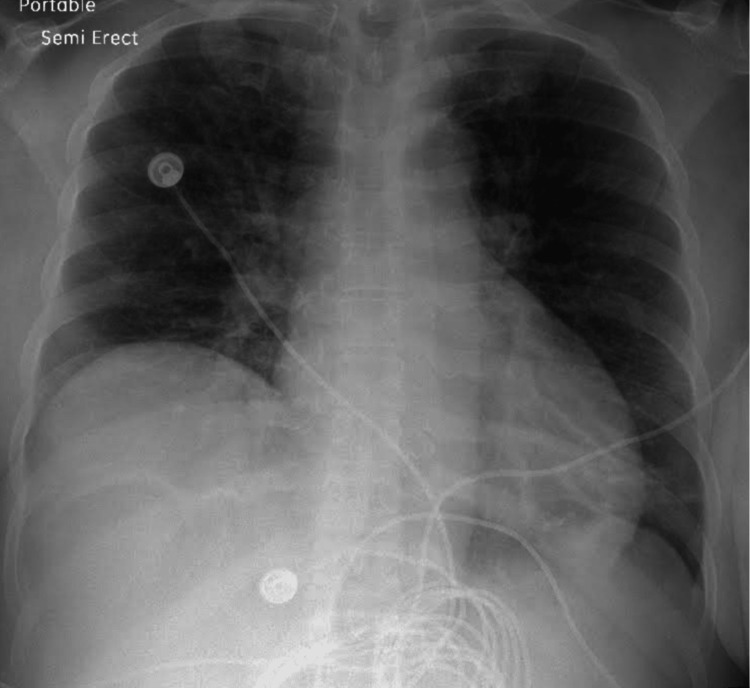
The initial chest x-ray depicting right upper lobar and diffuse left lung airspace opacities, possibly suggesting atypical pneumonia.
